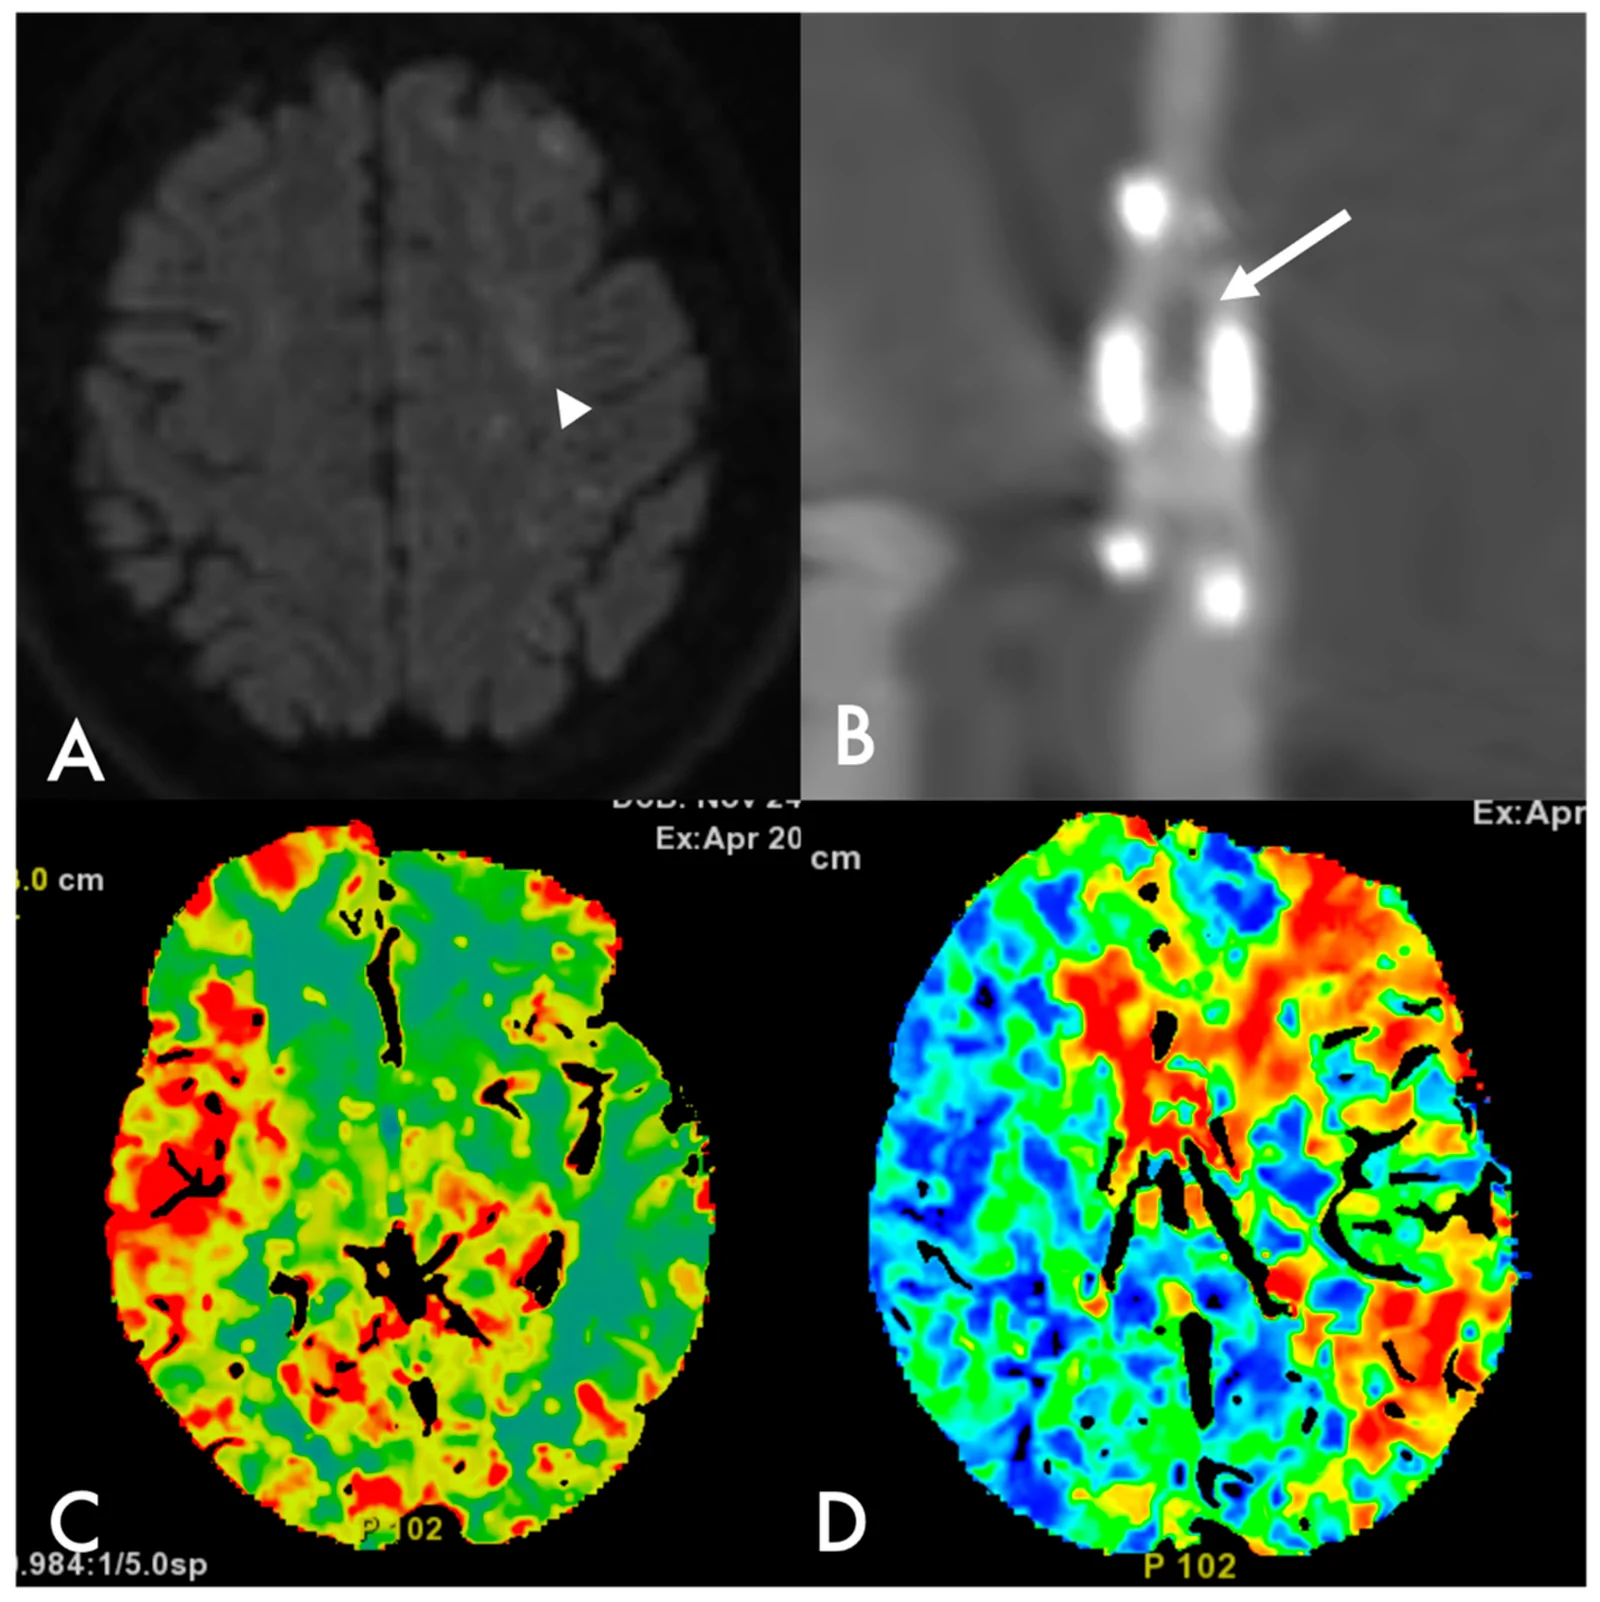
Diffusion MRI performed on postoperative day 5 shows areas of signal restriction compatible with recent ischemia (arrowhead) (A). DE-CTA with iodine map and stent reconstruction demonstrates thrombosis in its distal portion (arrow) (B). CTP maps show reduced CBF in the left hemisphere (C) and prolonged MTT in the watershed ACA-MCA territory (D).
